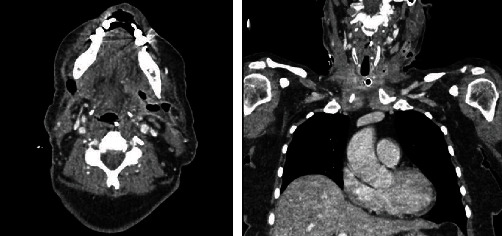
Final CT neck and chest 01/03/2021.
